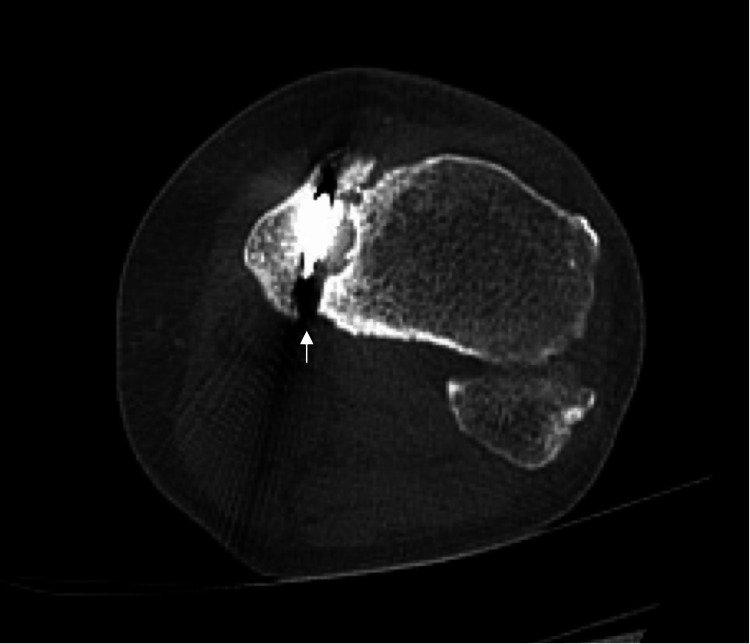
Computed tomography of the peri-implant fracture
